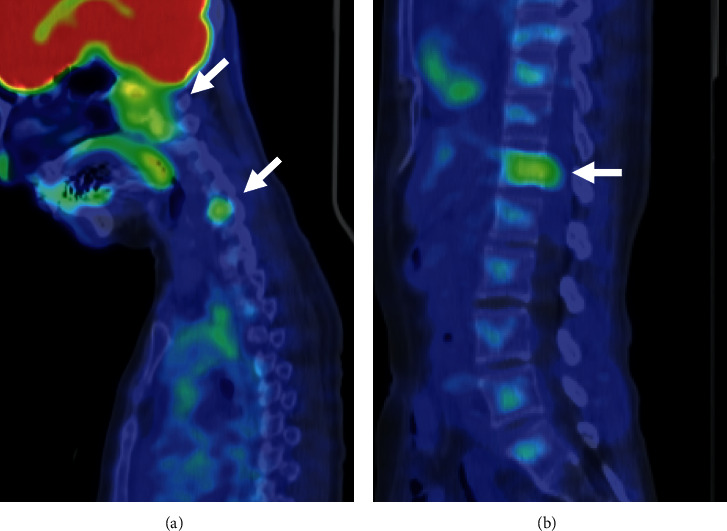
^18^F-FDG-PET/CT scan at first presentation. ^18^FDG uptake in (a) skull base and C6 and (b) T12.
